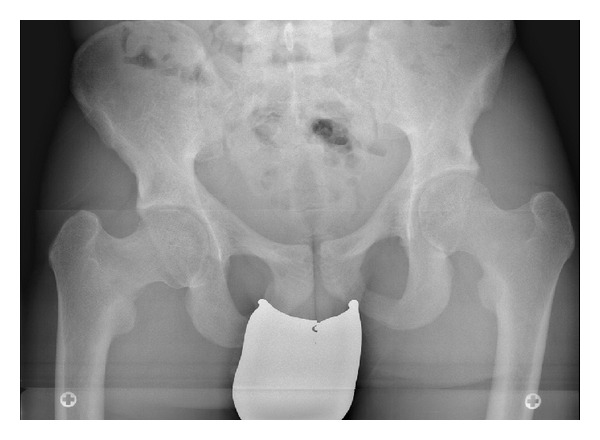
Standard pelvic radiograph taken 4 years ago showing no radiographic evidence of a fracture.
